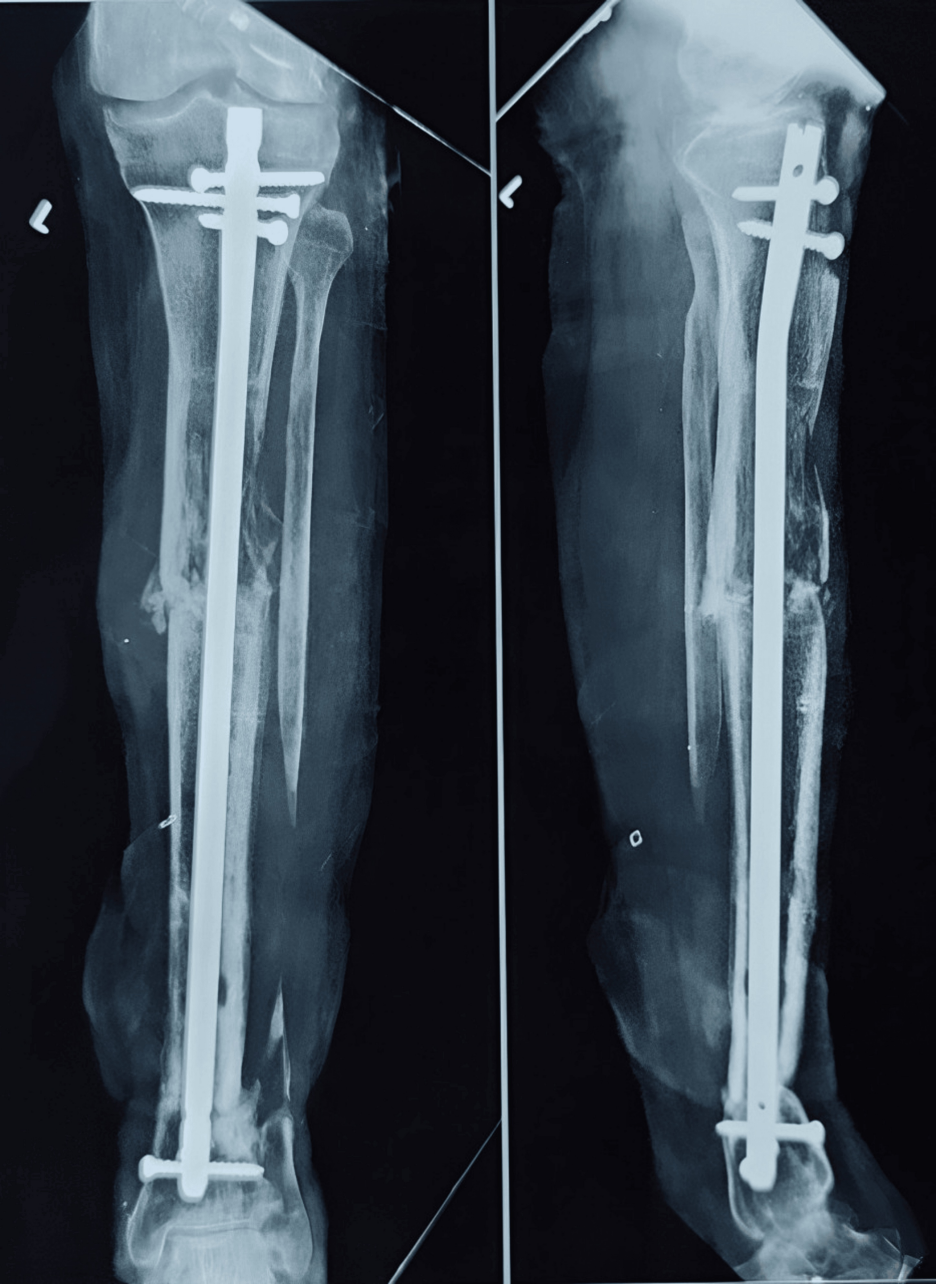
Postoperative X-ray showing the interlocking nail
